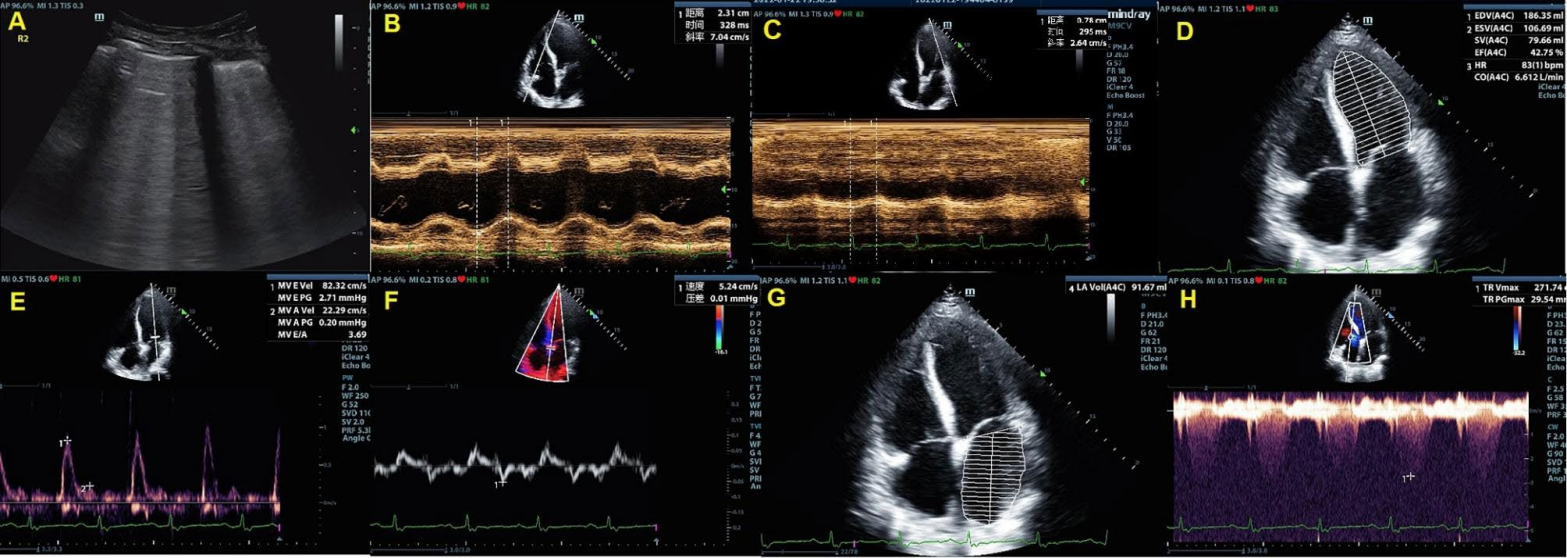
Lung ultrasound and echocardiographic examination. A. Lung ultrasound showing diffuse B-lines; B. TAPSE measurement; C. MAPSE measurement; D. LVEF measurement; E. Mitral E peak velocity; F. Septal e’ measurement; G. LA volume measurement; 1 H. TR measurement TAPSE: tricuspid annular plane systolic excursion; MAPSE: mitral annular plane systolic excursion; LVEF: left ventricular ejection fraction; LA: left atrium; TR: tricuspid regurgitation
